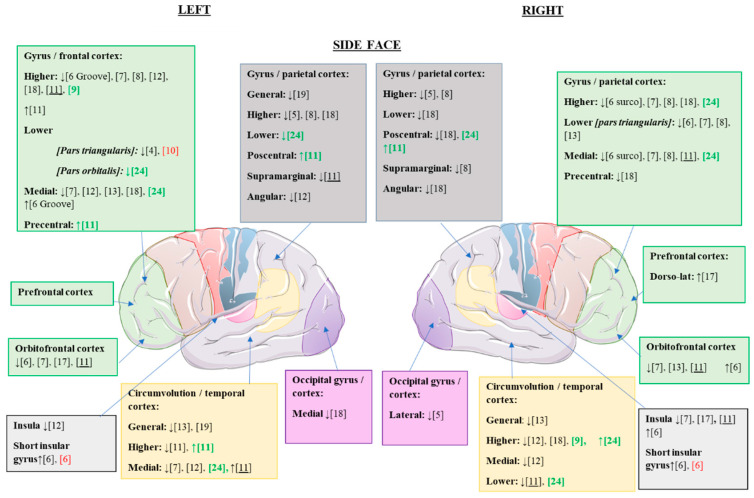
Statistically significant cortical structural alterations in GMV and CT in the different eating disorders compared to control values; lateral view. ↑, increased GMV/CT; ↓, reduced GMV/CT; citations in black, changes observed in patients with AN versus controls; citations in red, changes observed in patients with REC versus controls (possible trait and nonstate changes); citations in green, changes observed in patients with BN versus controls; underlined citations, changes observed in patients with AN versus REC.
